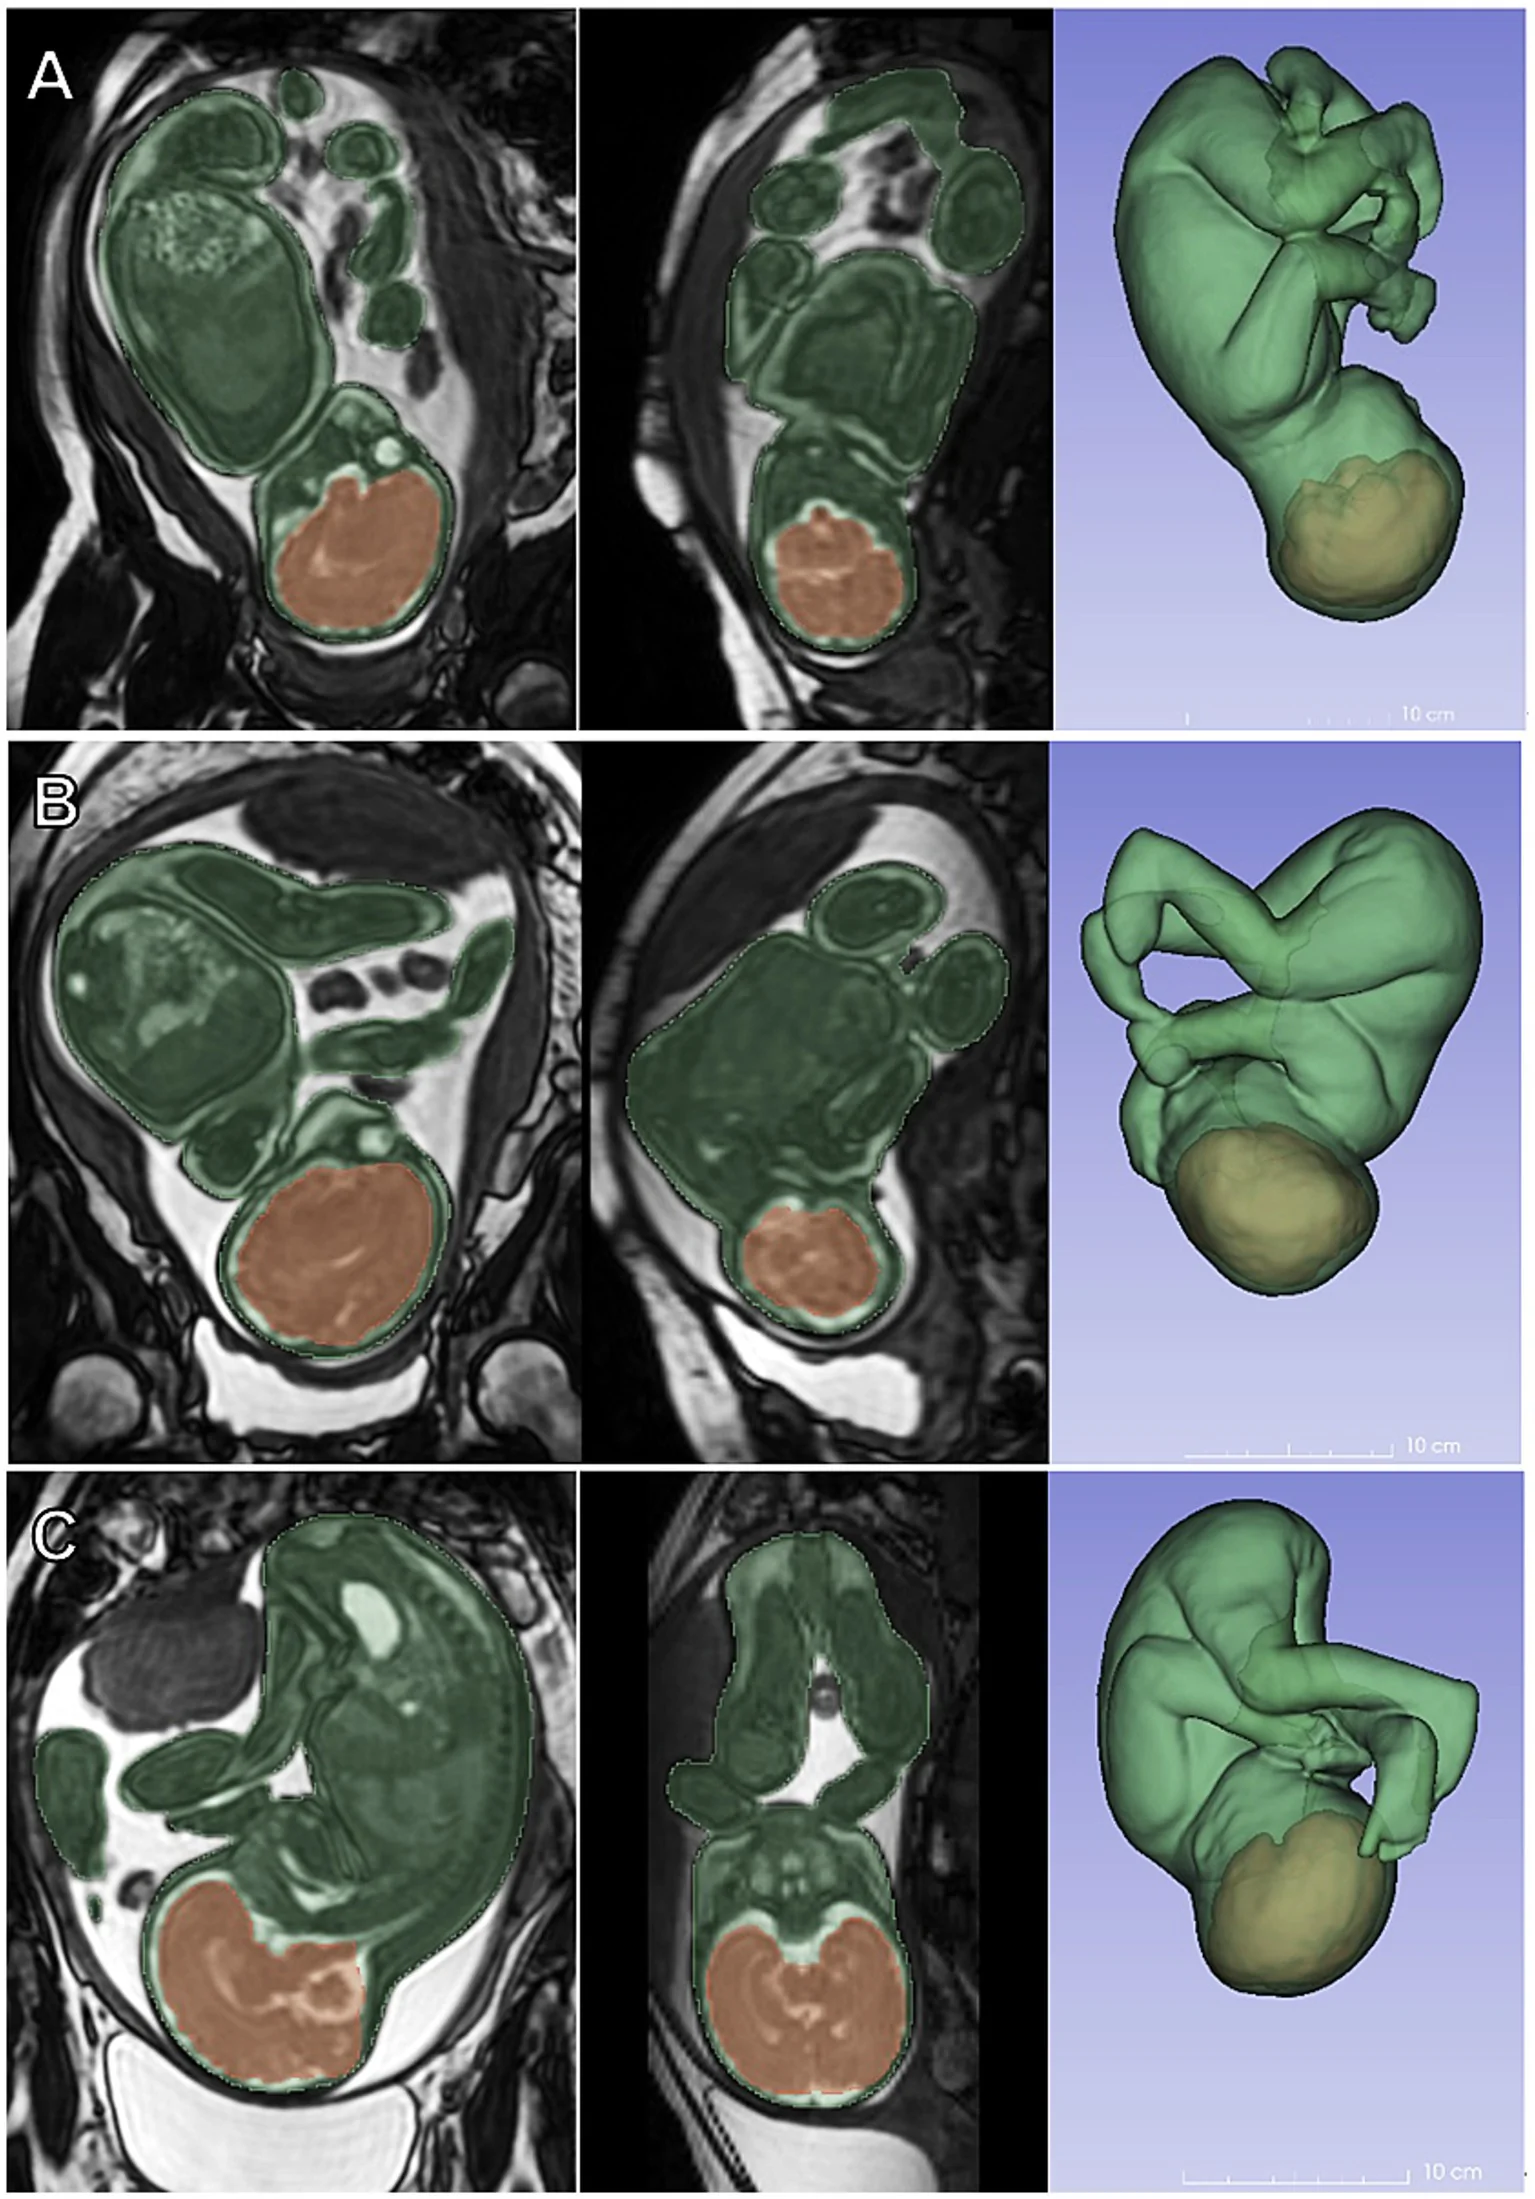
Fetal MR images, masks, and 3D segmentation visualization. (A) Normal case: volume ratio = 0.116; (B) SGA case: volume ratio = 0.128; (C) FGR case: volume ratio = 0.142.
